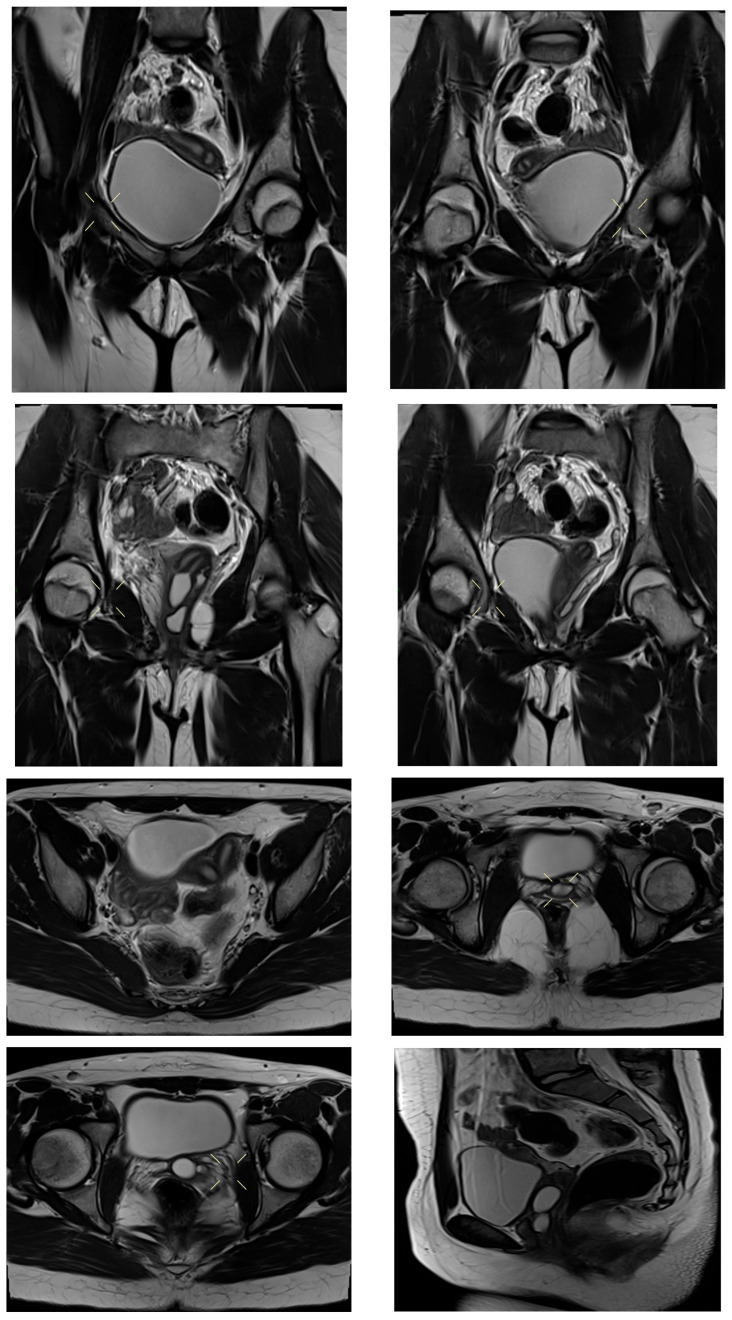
Follow-up MRI pelvis in Case 4 confirming persistence of left-sided vaginal cystic structures with ureteral communication and no evidence of hematocolpos or hematometrocolpos at the time of imaging.
